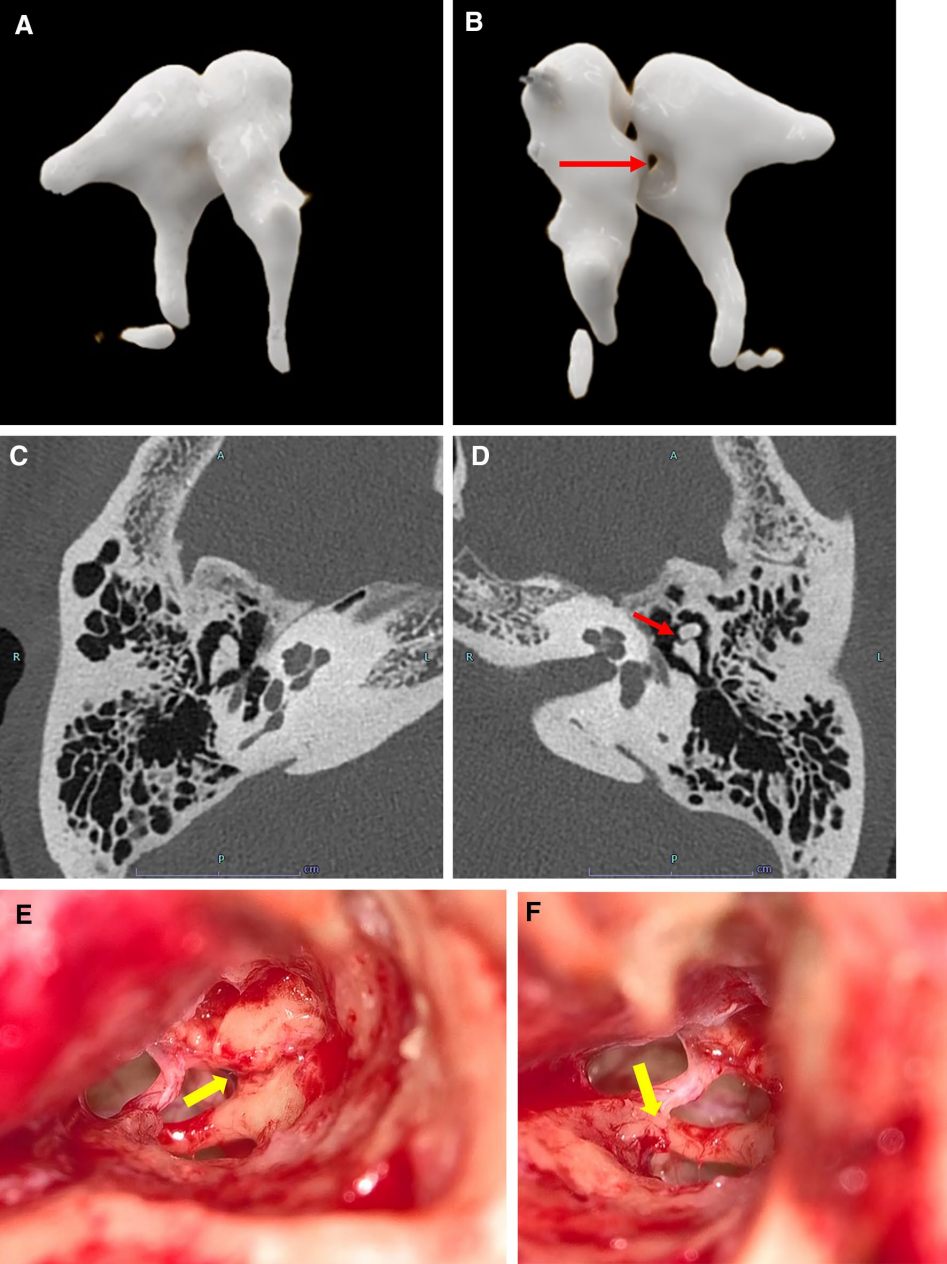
A case of traumatic ossicular chain dislocation in a woman in her 50 s with a chief complaint of left hearing loss. A; 3D image of the normal right ossicles. B; 3D image of left ossicular chain dislocation. C; Axial image of the normal right ossicle. D; Axial image of left ossicular chain dislocation. E, F; intraoperative images. PCD-CT showing dislocation of the left incudomalleolar joint in the 3D image (B, arrow) and axial image (D, arrow). The head of malleus is anteriorly dislocated in the left tympanum, unlike the normal incudomalleolar joint in the right (A, C). During the surgery, anterior deviation of the malleus was observed. Dislocation of the incudomalleolar joint (E, arrow). There was no evidence of incudostapedial joint disarticulation (F, arrow). Preoperative CT findings were consistent with intraoperative findings.
